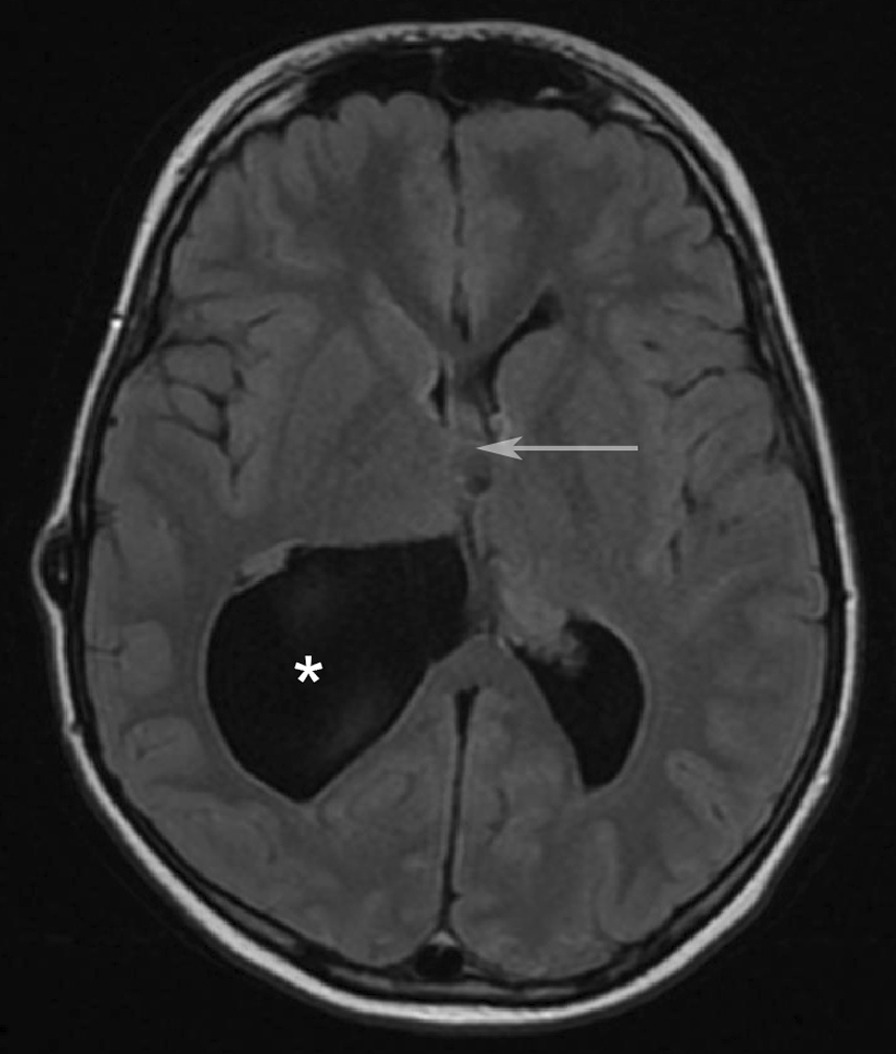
T1-weighted axial MRI obtained at age 16 years, showing occlusion of the foramen of Monro by a colloid cyst (arrow) and a cystic enlargement of the posterior horn of the right lateral ventricle. * = lateral ventricle
